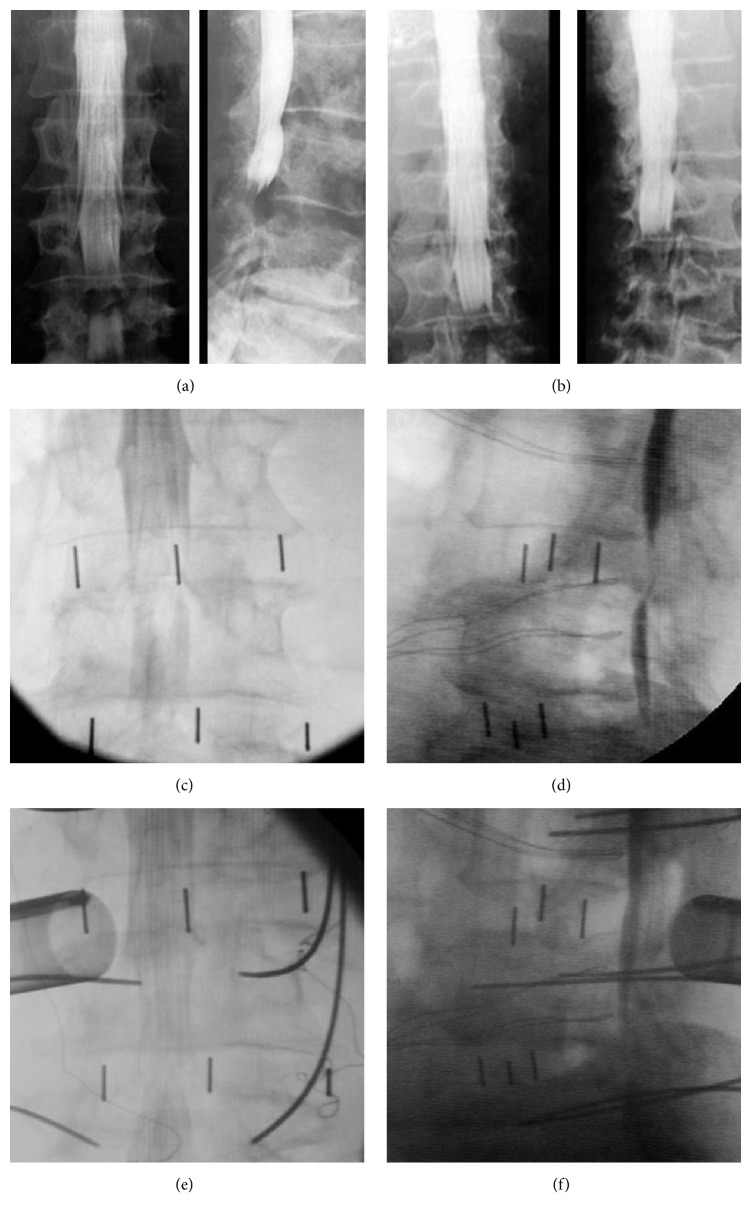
Radiographs of Case (C)*: preoperative myelography identified filling defects of both L3-4 and L4-5 levels ((a) and (b)). Intraoperative myelography did not reveal adequate indirect canal decompression at L3-4 level ((c) and (d)). Sufficient canal decompression was achieved after same-level MECD ((e) and (f)).
